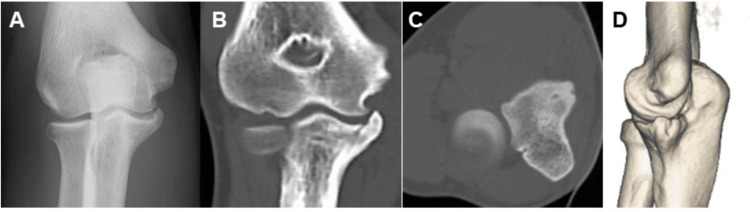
Postoperative radiographic and CT findings of case 1 A. Postoperative anteroposterior radiograph showing satisfactory realignment of the fragment following suture bridge fixation. B. Coronal CT image. D. Three-dimensional CT reconstruction demonstrating successful bone union.
